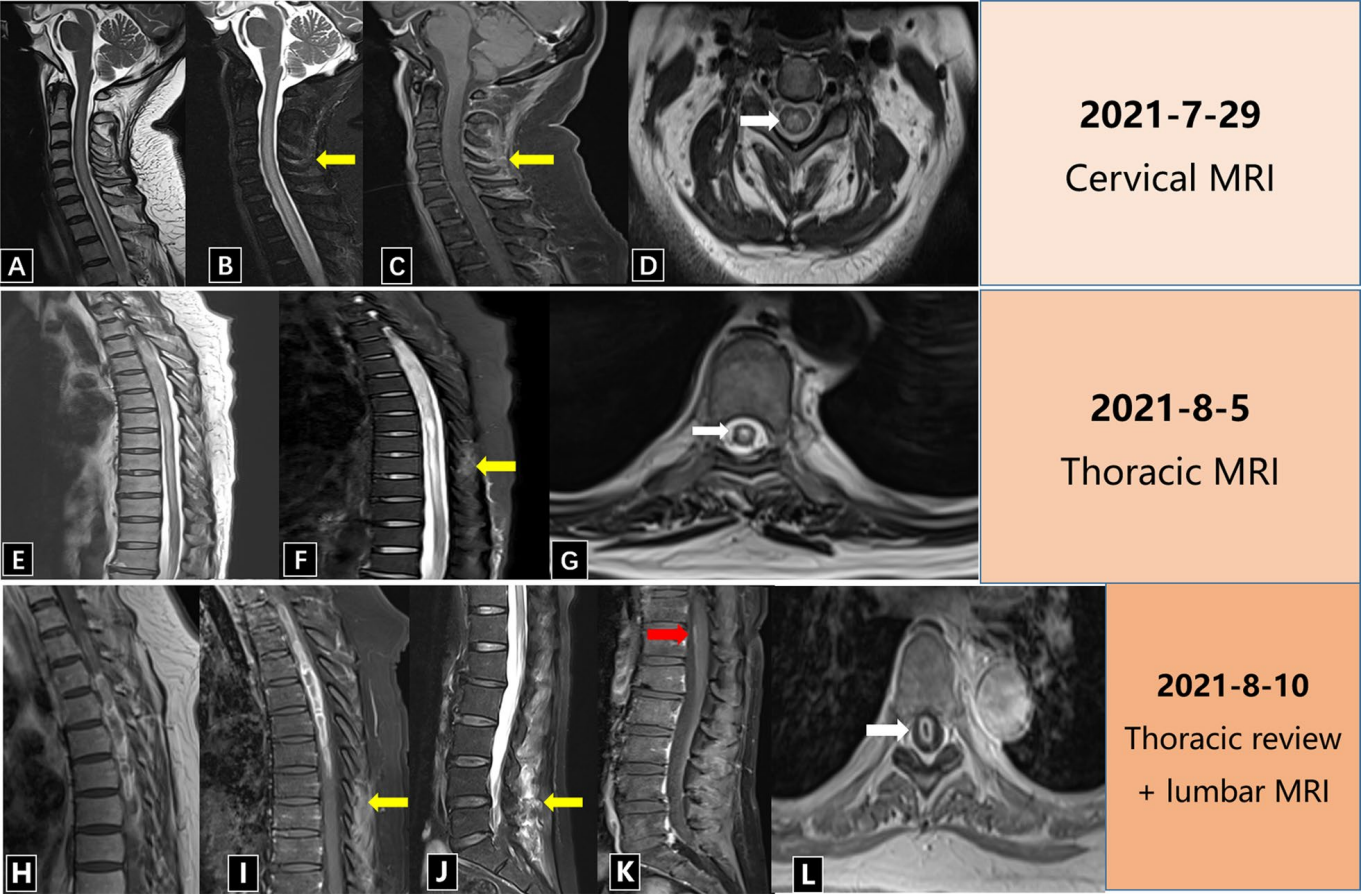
The patient’s cervical, thoracic and lumbar spine MRI. A–D are the cervical spine MRI on July 29, 2021. A is T2WI, and B is the T2 fat suppression sequence. It shows hyperintensity in the entire cervical spinal cord. The posterior soft tissue shows patchy hyperintensity as yellow arrow. C (T1WI enhanced sequence) shows the posterior soft tissue obviously enhanced in patches as yellow arrow. D (axial position of the cervical spine of T2WI) shows high signal in the spinal cord as white arrow. E–G is the thoracic MRI on August 5, 2021. E is T2WI, F is the T2 fat suppression sequence, showing the entire thoracic spinal cord with high signal intensity, and the yellow arrow shows the posterior soft tissue with patchy high signal. G shows the axial T2WI of the thoracic spine with high signal in the thoracic spinal cord as white arrow. H–L is the thoracic follow-up MRI and lumbar spine MRI on August 10, 2021. H (Thoracic spine of coronal T2WI) compared with the previous image (E), there is a cavity in the center of the thoracic spinal. I (T1WI enhancement), the intrathoracic spinal cord lesion shows a long strip-shaped edge enhancement, and the posterior soft tissue shows patch-like enhancement. J (T2WI fat suppression sequence of the lumbar spine) shows the posterior soft tissue with patchy hyperintensity. K (coronal enhanced sequence) shows the streak-shaped spinal membrane enhancement as red arrow. L is the axial position, showing the ring-shaped enhancement high signal in the thoracic spinal cord as white arrow
